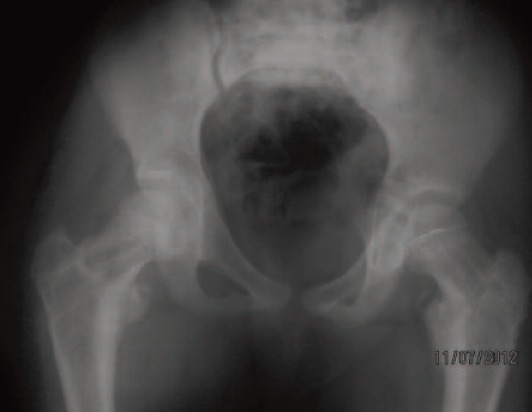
Anterioposterior radiograph of pelvis immediately after
